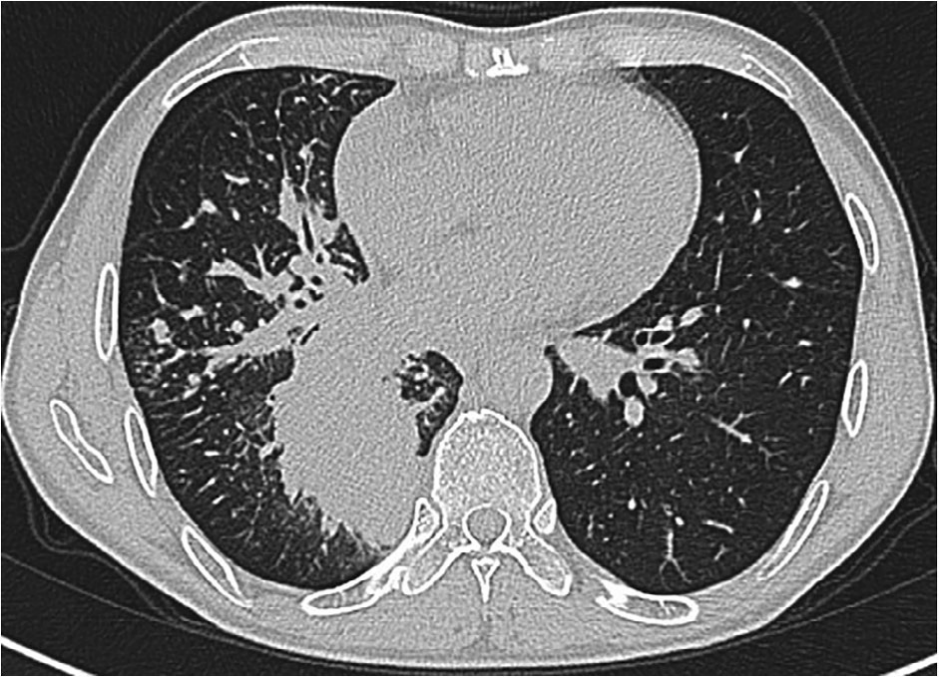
Chest computed tomography (CT) scan revealed an 5.8 × 4.4 cm mass in the right inferior lobe of patient No. 1.
